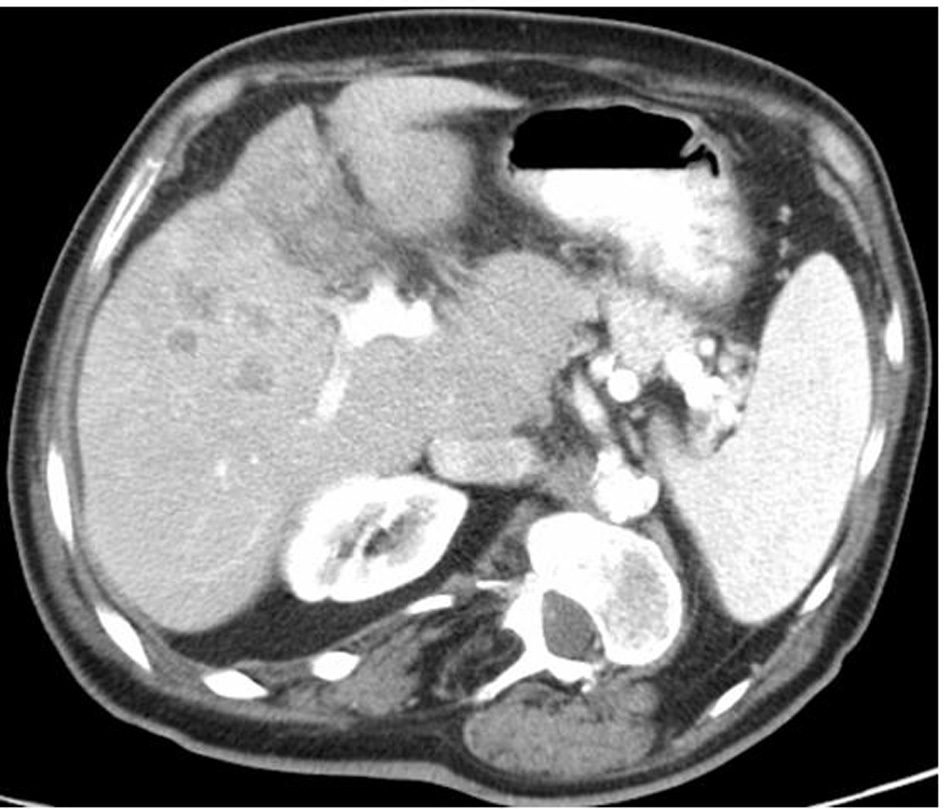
Abdominal computed tomography (CT) findings. Scan revealed several metastatic lesion in the liver parenchyma.
